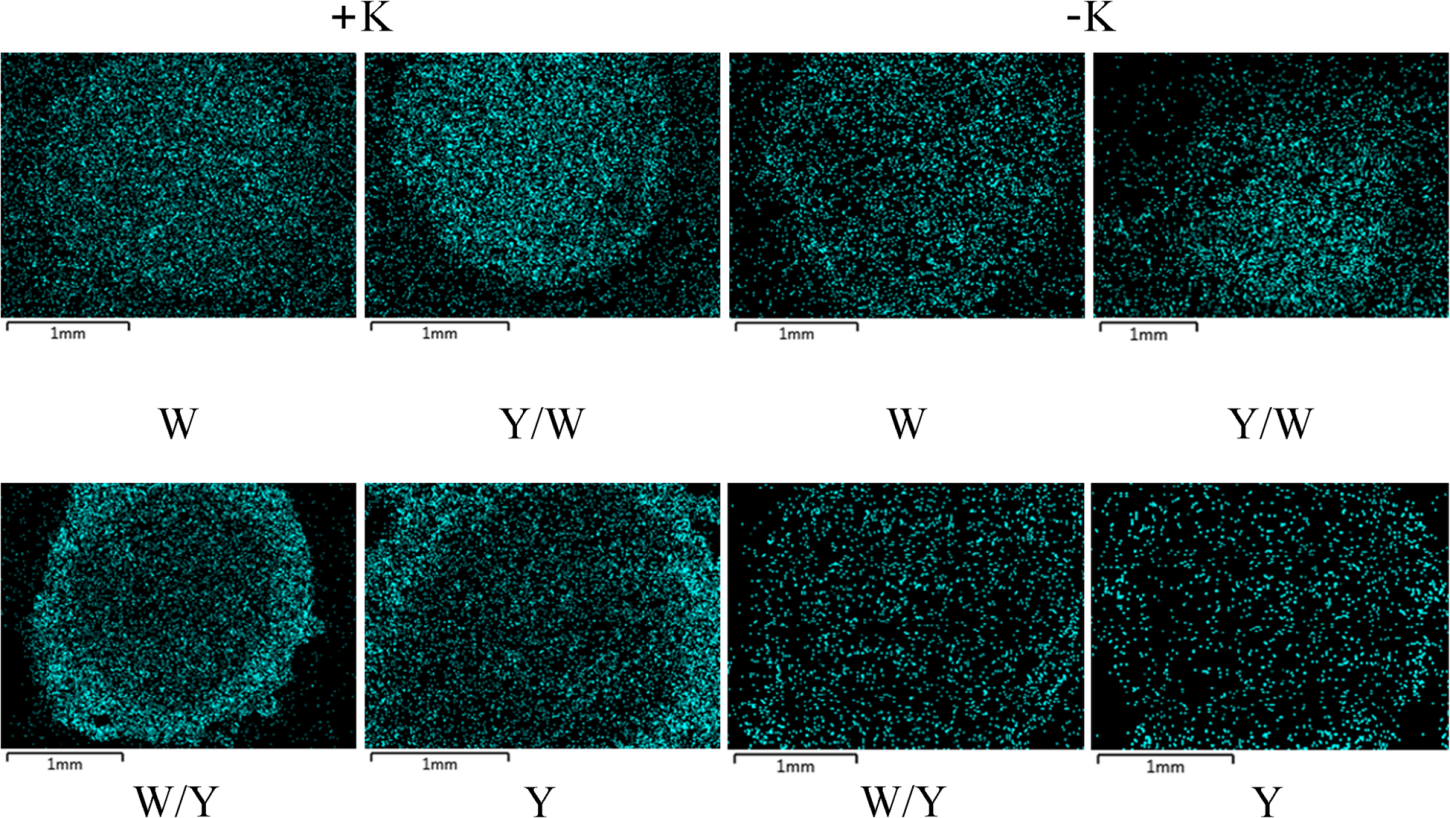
Images of the K^+^ distribution in tobacco roots from the X-ray microanalysis. Blue particles represent the charge of K^+^. The tobacco graft combinations included the ungrafted tobacco W (Wufeng No.2) and Y (Yunyan 87), and grafted tobacco Y/W (Y grafted onto W) and W/Y (W grafted onto Y)
